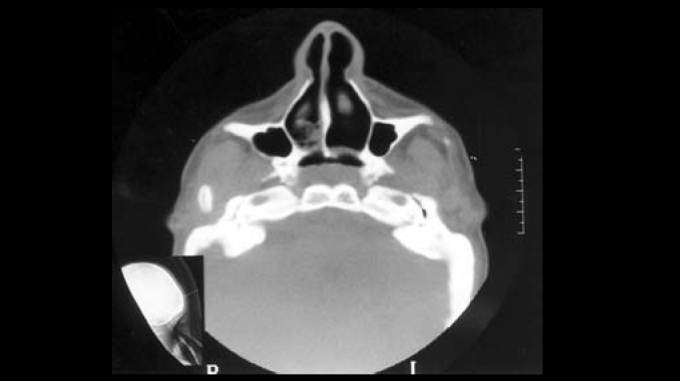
CT scan showing choanal atresia (predominance of bone tissue on the left lamina) –axial section.
